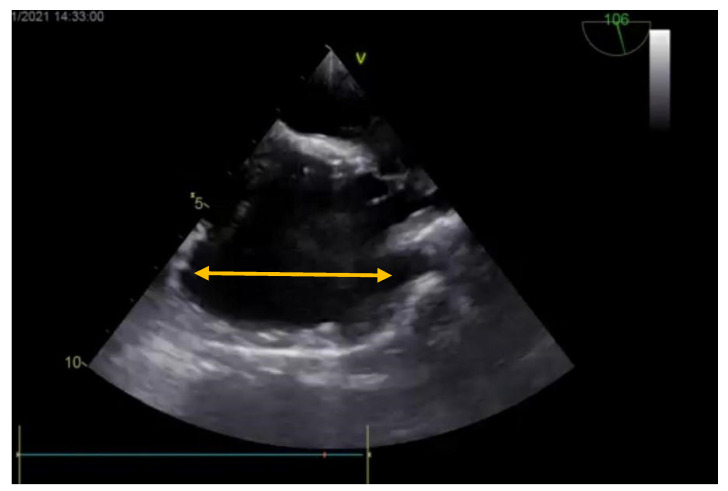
Postoperative echocardiography (bicaval view) showing the closed ASD and the plasty enlarging the SVC and right atrium (yellow arrow).
